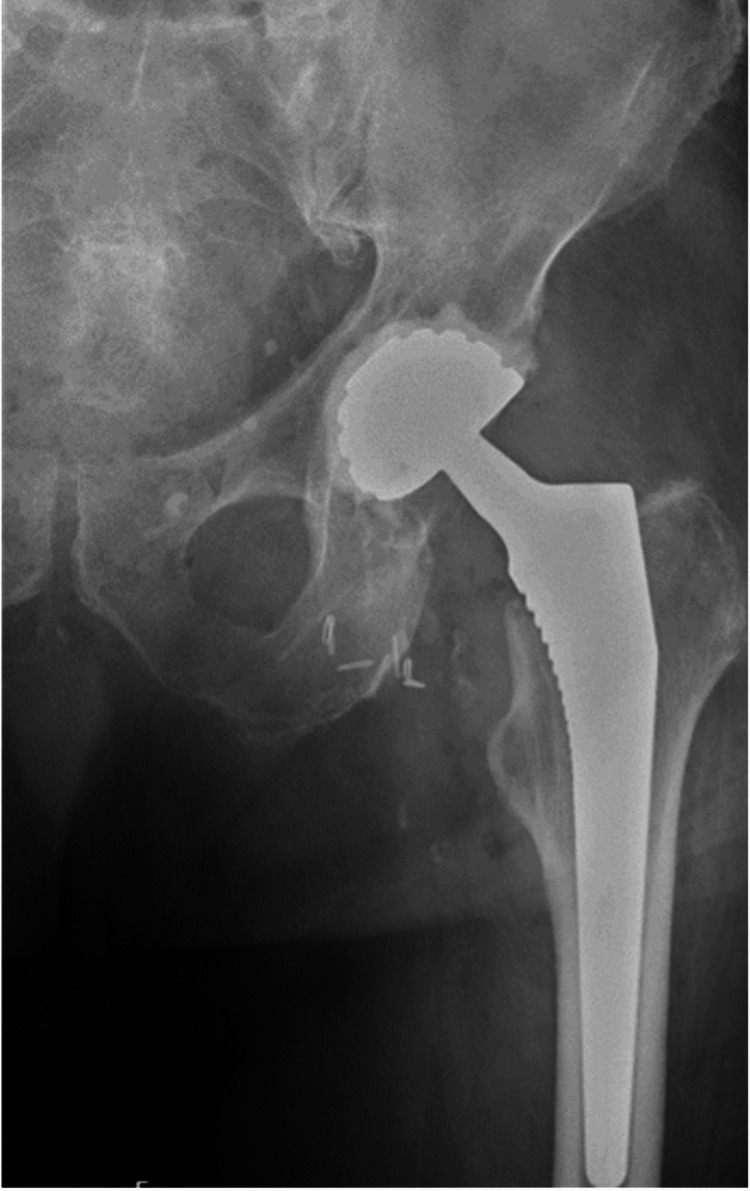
Hip radiograph after revision surgery with cemented dual-mobility acetabular component.
